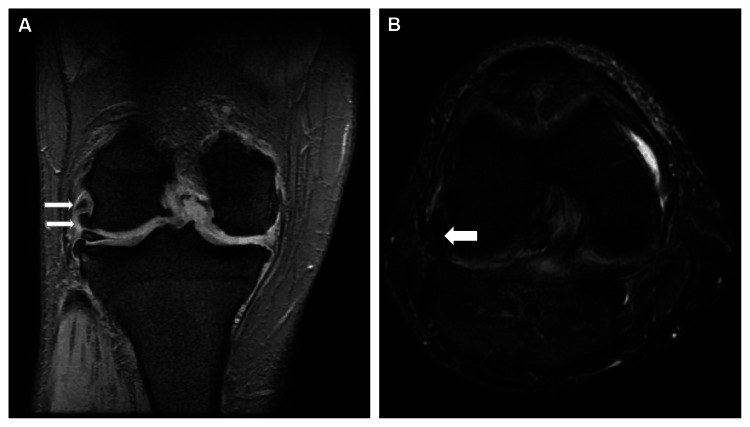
Postoperative MRI images of the right knee. (A) Coronal MRI slice. The upper white arrow indicates the popliteal tendon, which was impinged by the osteophyte, whereas the lower white arrow indicates the osteophyte on the lateral femoral condyle. (B) Axial MRI slice. The arrow indicates the osteophyte on the lateral femoral condyle, located immediately next to the popliteal tendon. LFC: lateral femoral condyle
